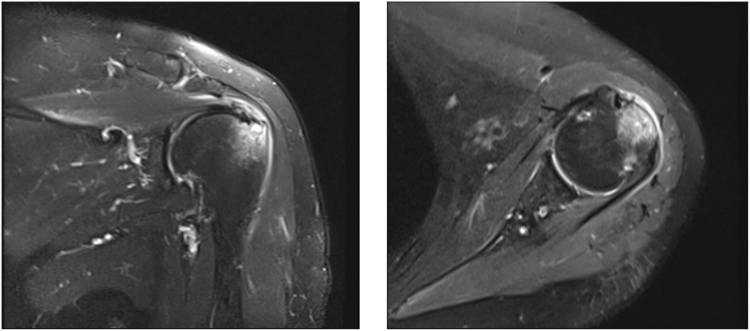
Pre-operative MRI of the left shoulder showing a high-grade partial-thickness bursal-sided supraspinatus tear and subacromial-subdeltoid bursitis. *MRI*, magnetic resonance imaging.
